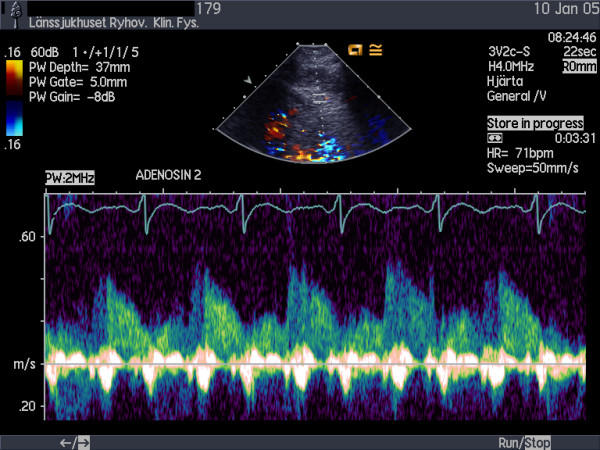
Spectral Doppler recording of LAD flow during stress.
